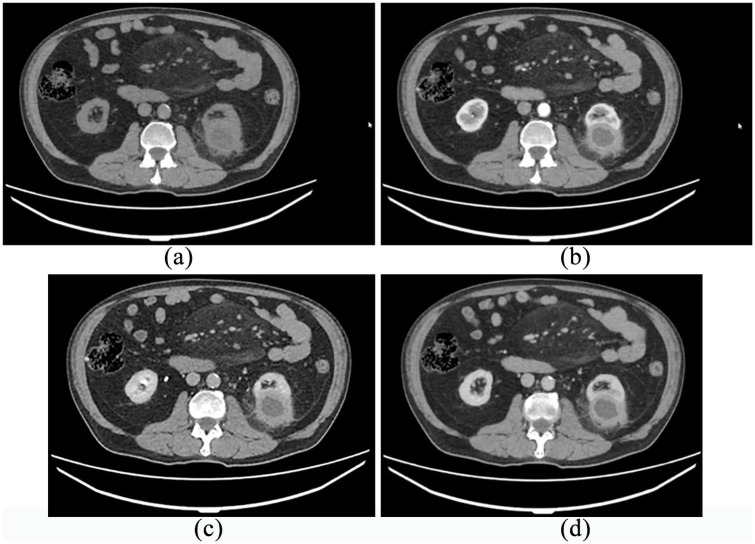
Abdominal CT scan: (a) no contrast, (b) arteriosus phase, (c) venous phase, and (d) excretory phase.
